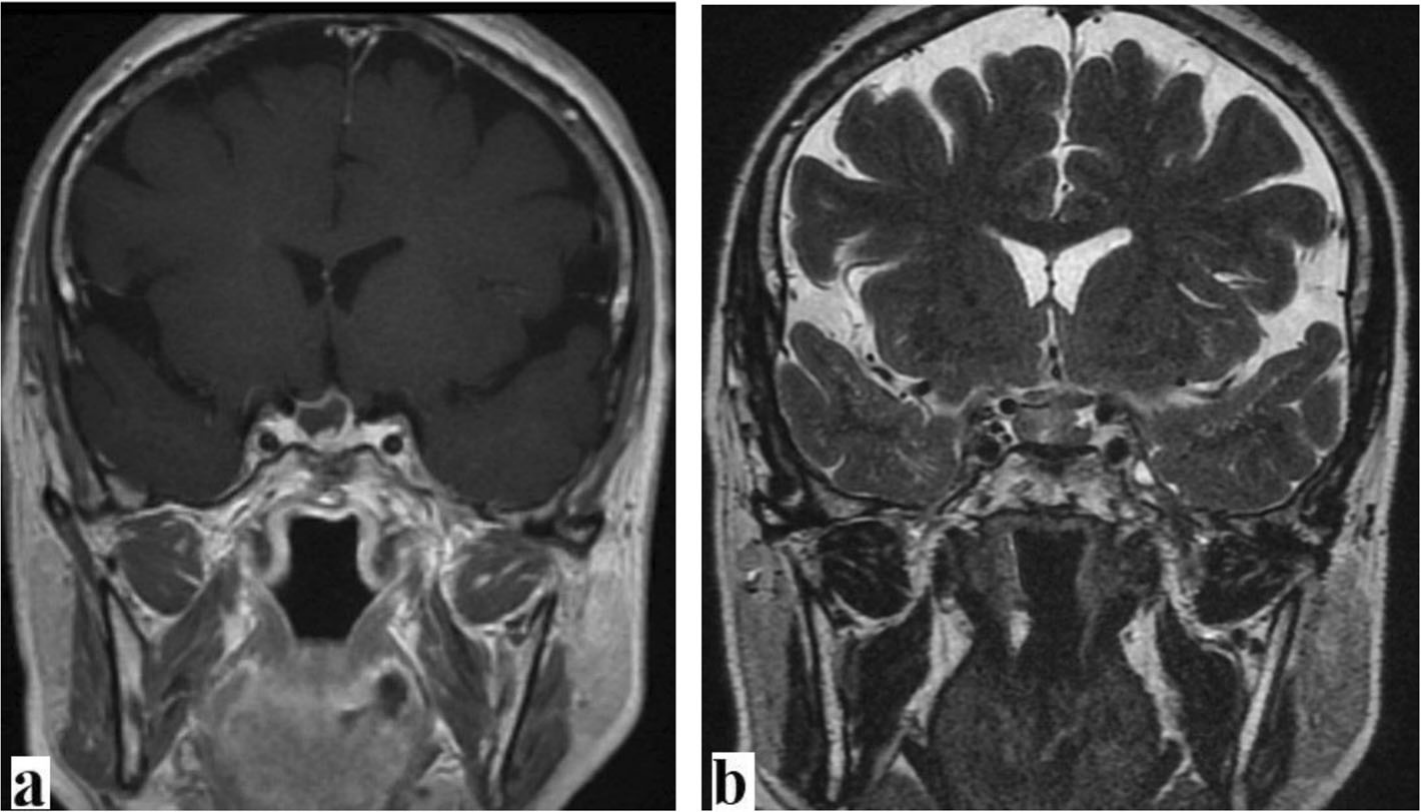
Same patient as in Fig. 1. Follow-up magnetic resonance imaging 3 months after the treatment showed a very sizable reduction of the pituitary abscess with disappearance of the peripheral edema and the local compression effect. a T1-weighted image + gadolinium. b T2-weighted image
